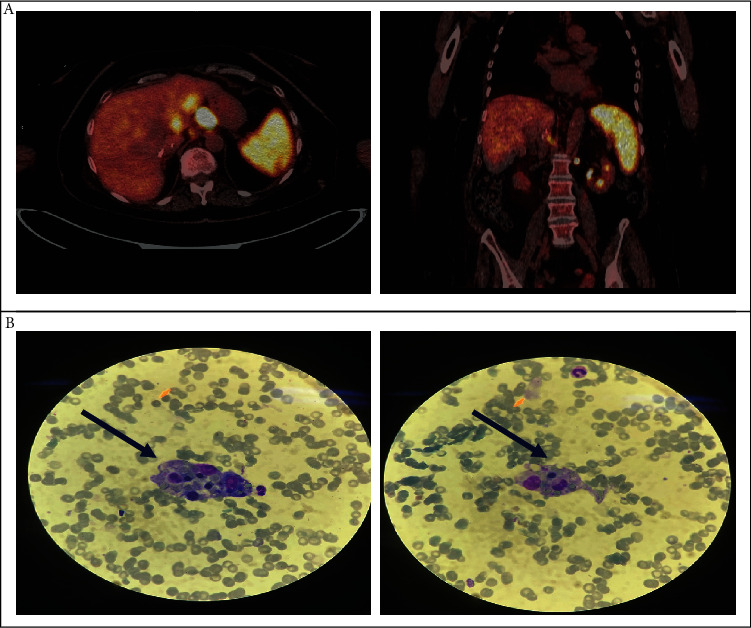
Pretreatment: (a) PET-CT: splenomegaly and increased metabolism in the spleen are observed at diagnosis; (b) bone marrow biopsy: macrophage phagocytosing erythrocytes and neutrophils (hemophagocyte).
